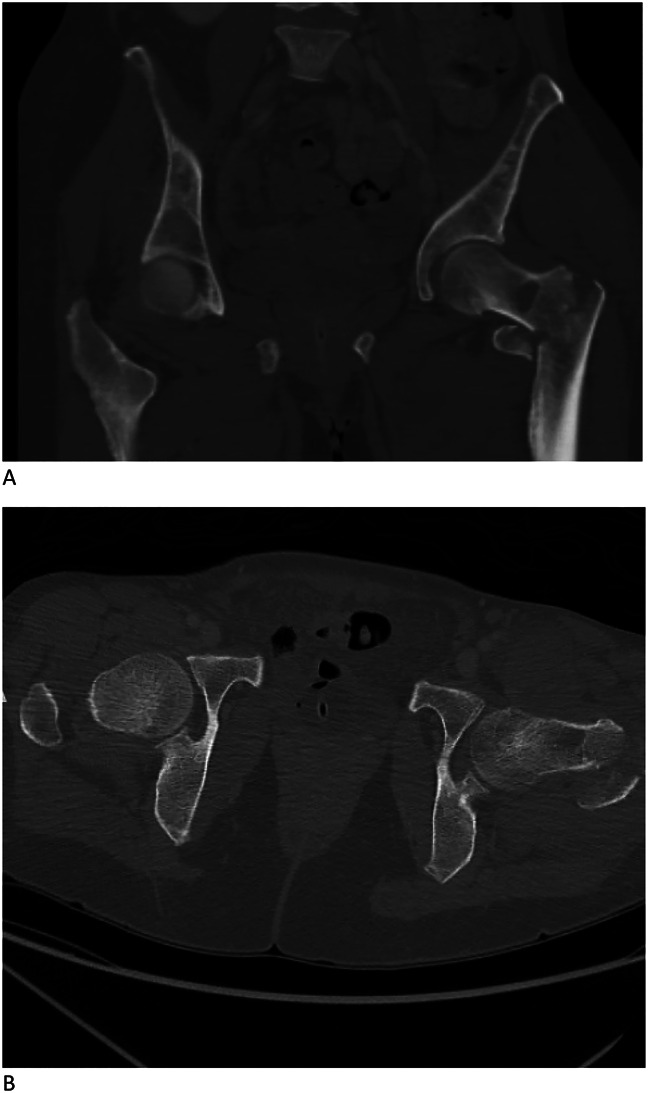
A and B, Coronal CT imaging revealed multiple lytic lesions in the pelvis (A). Axial CT imaging shows an intertrochanteric fracture of the left hip and further demonstrates lytic lesion of the left femur (B).
